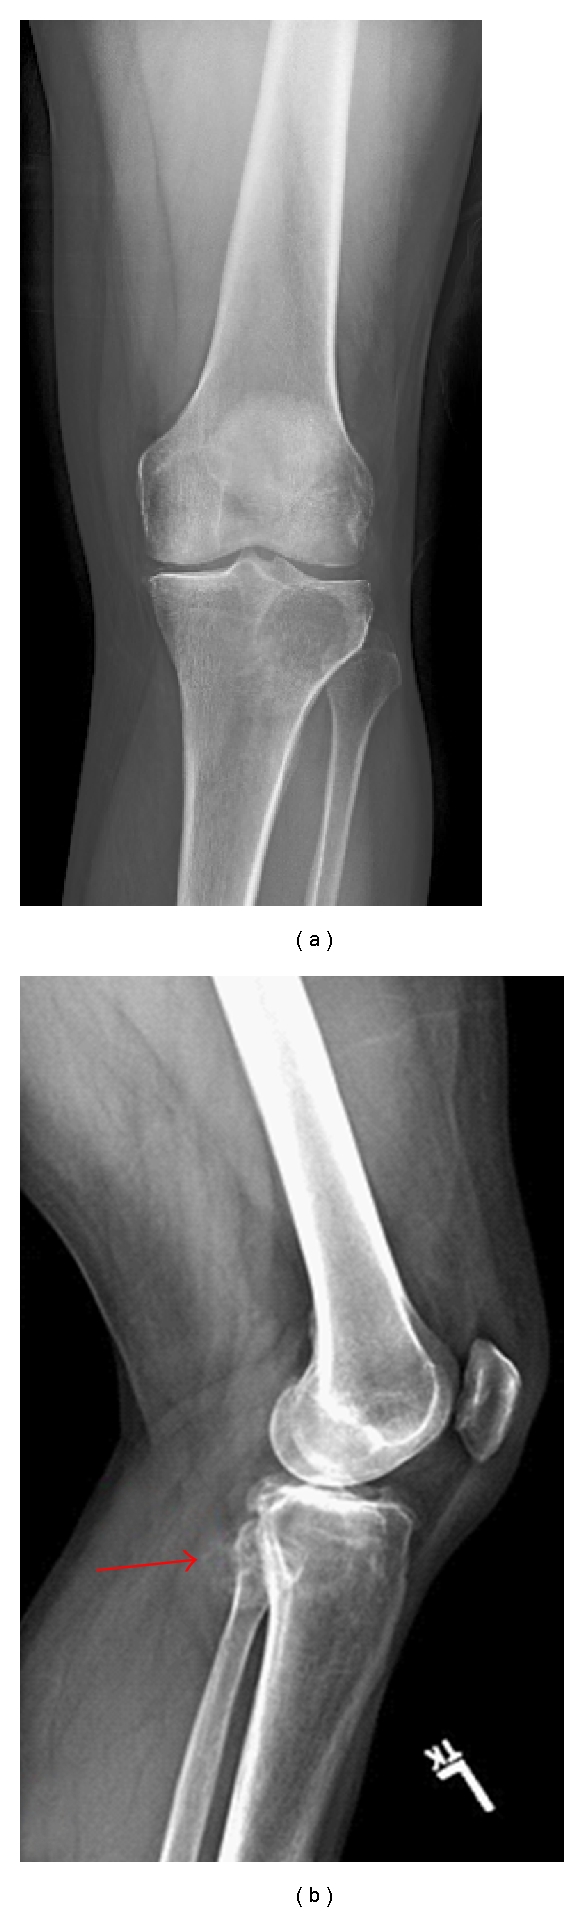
(a) A/p radiograph of the left knee shows a round lucency at the lateral tibial plateau with well-defined proximal margins and a less well-defined distal margin.(b) Lateral radiograph shows a stippled mineralization (arrow) projecting into the soft tissues posteriorly, measuring approximately 2.5 cm in maximal diameter.
